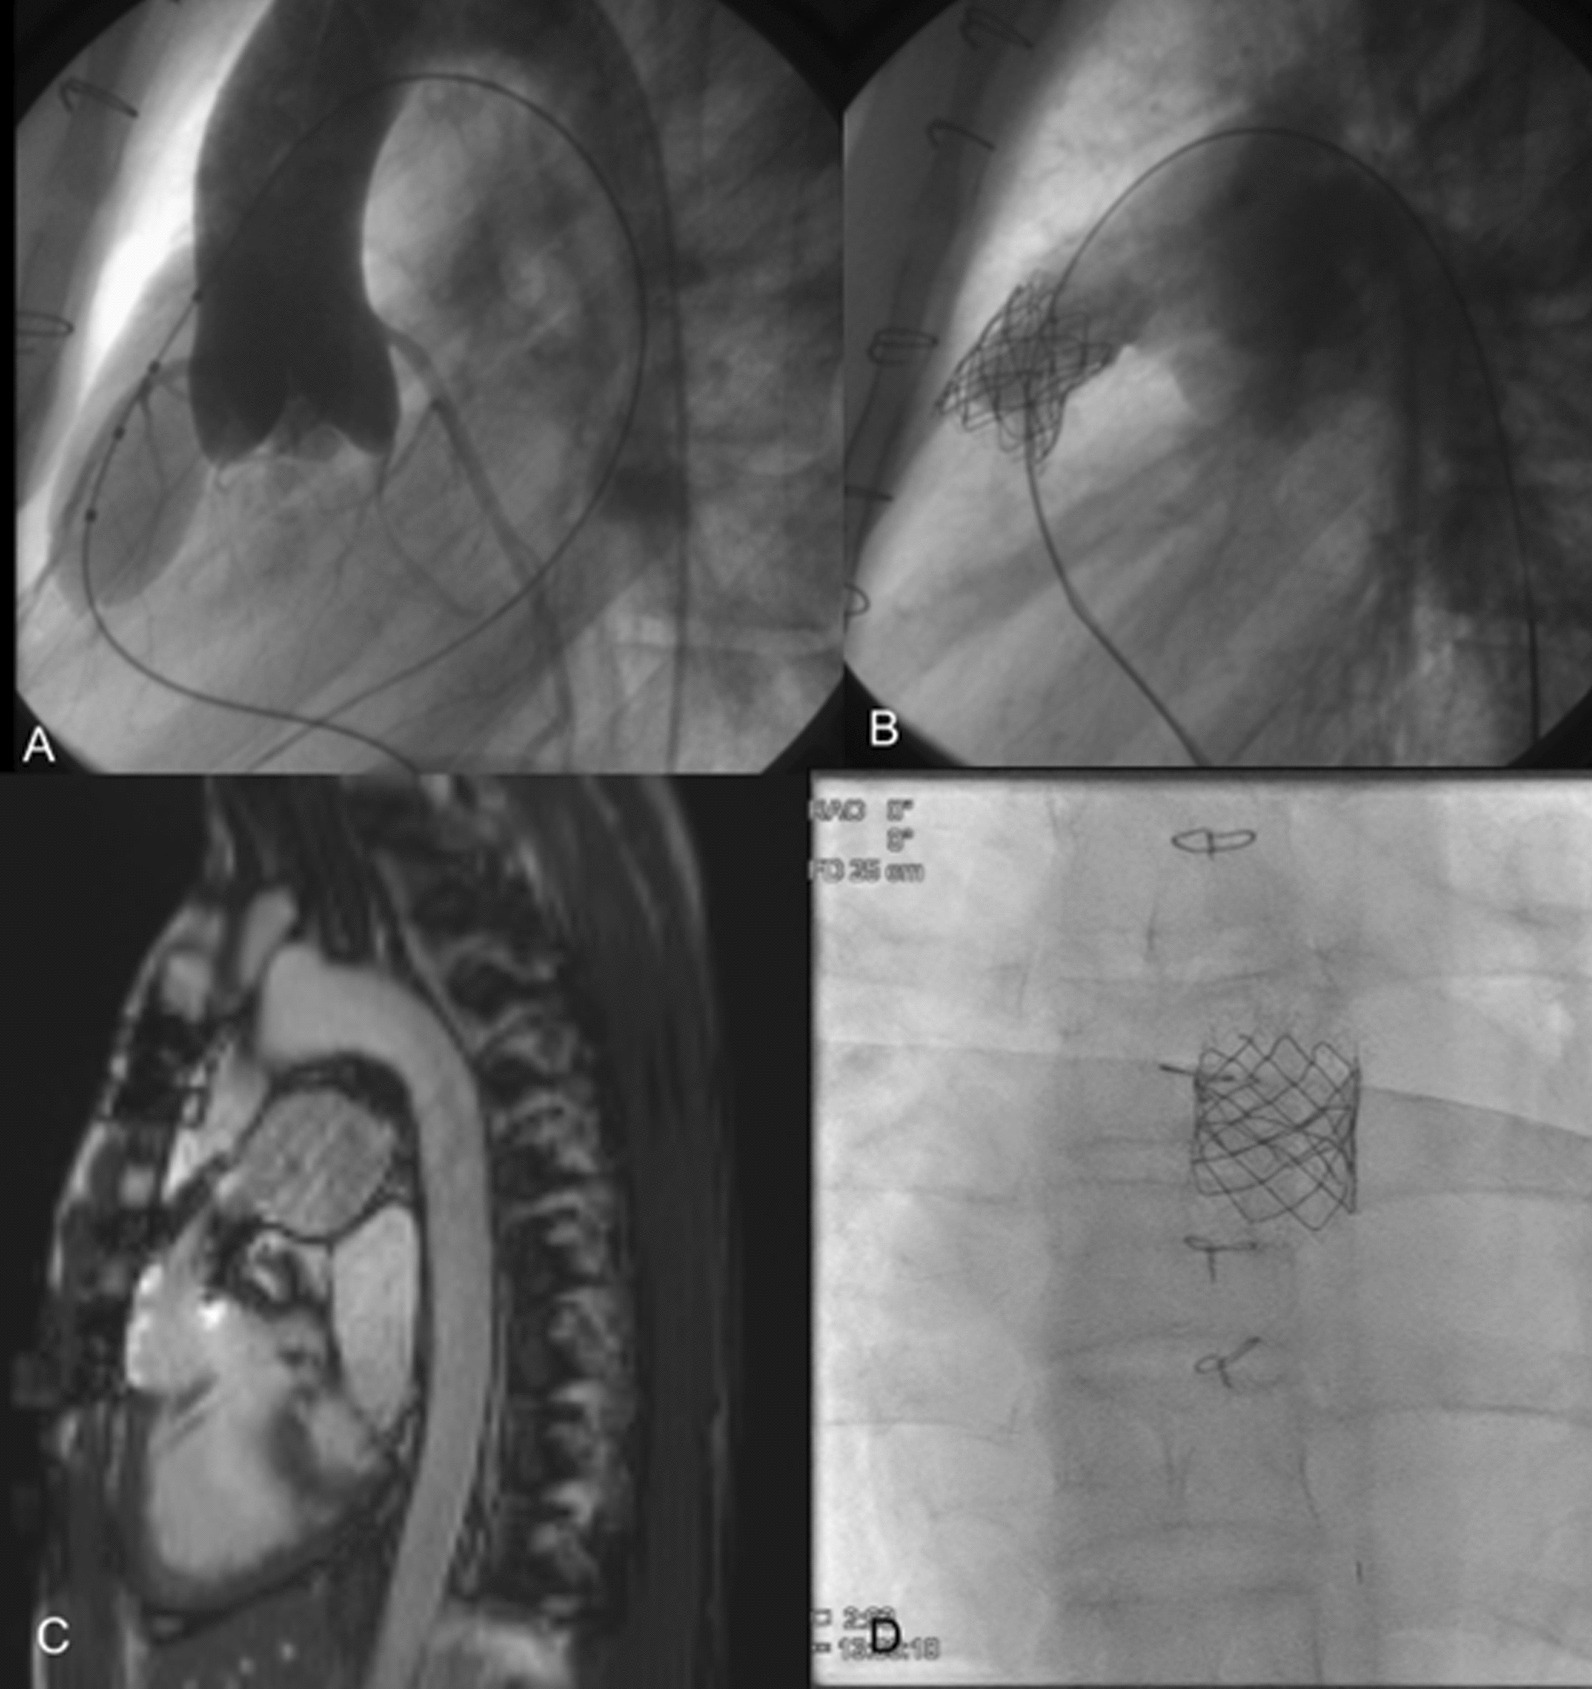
A Aortography performed during 20-mm Mullins-X balloon inflation in the potential valve landing zone in lateral projection shows no coronary artery compression. B Angiography performed after successful implantation of AndraStent and 18-mm Melody valve shows good effect of the procedure with trace of a regurgitation. C Magnetic resonance imaging performed 8 years after the procedure shows good performance of the conduit with no regurgitation, no subpulmonary valve enlargement, and preserved subpulmonary ventricle function (EF of 48%). D Fluoroscopy 7 years after implantation of the Melody valve shows small, nonsignificant stent fractures with preserved stent and valve structure
